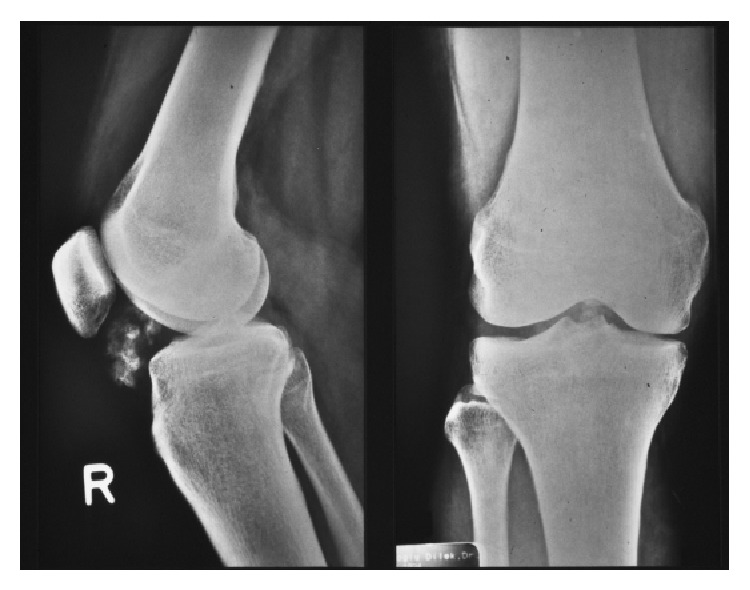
Plain radiography, anteroposterior and mediolateral view of the knee joint.
